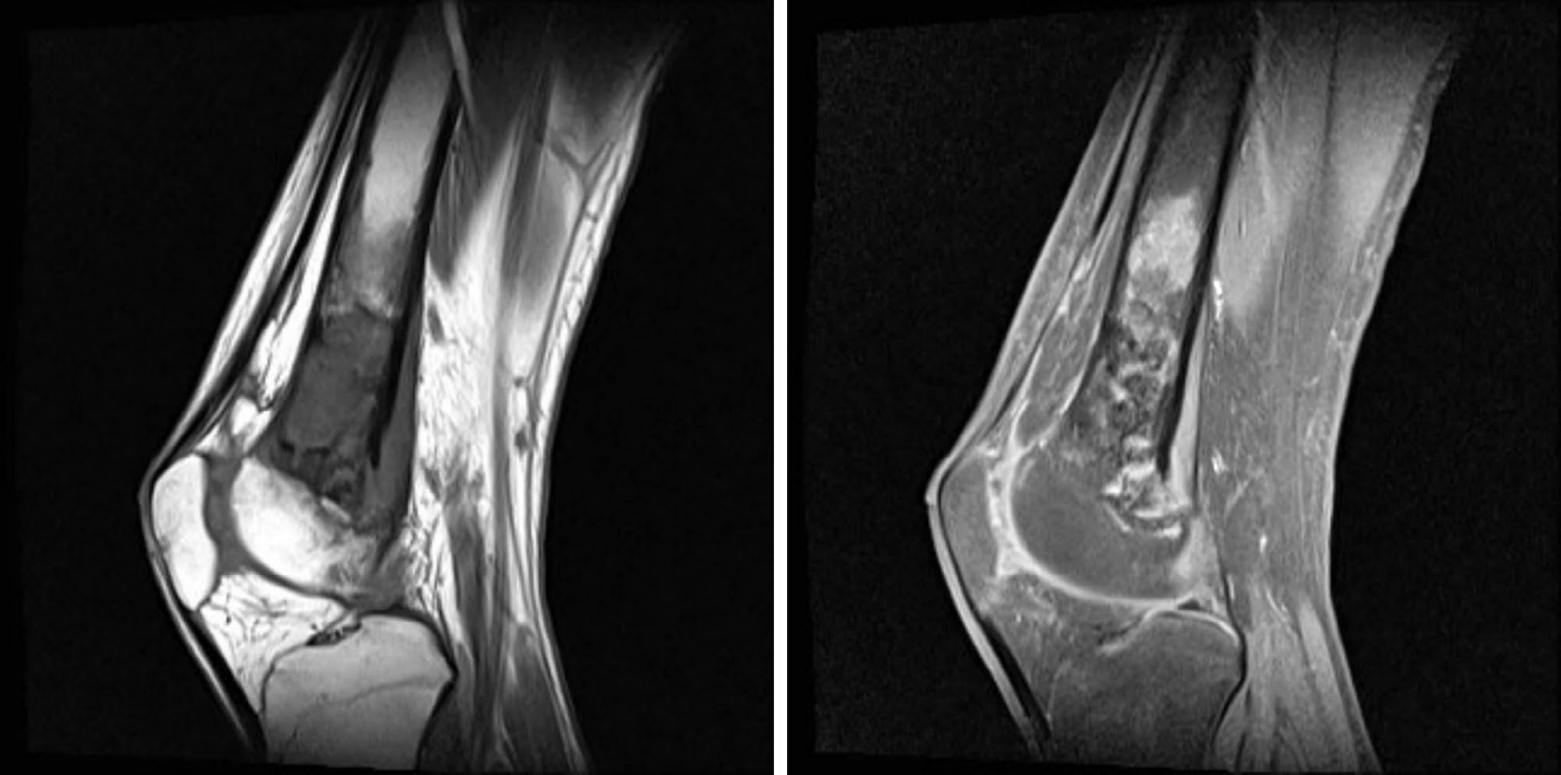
Control MRI after chemotherapy showing a clear reduction in the volume of the tumor process.
